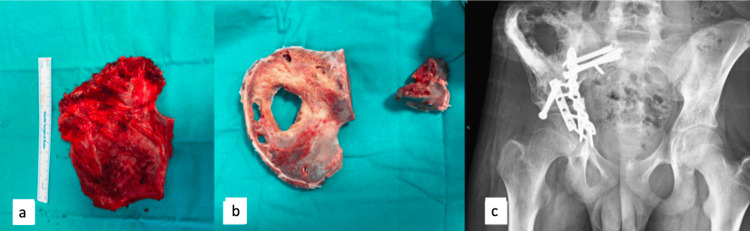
Clinical pictures of Ewing sarcoma patient with extracorporeal irradiation treatment; (a) clinical picture after tumor removal; (b) the prepared graft after bone is cleared from tumor and extracorporeal irradiation treatment; (c) post-operative x-ray
